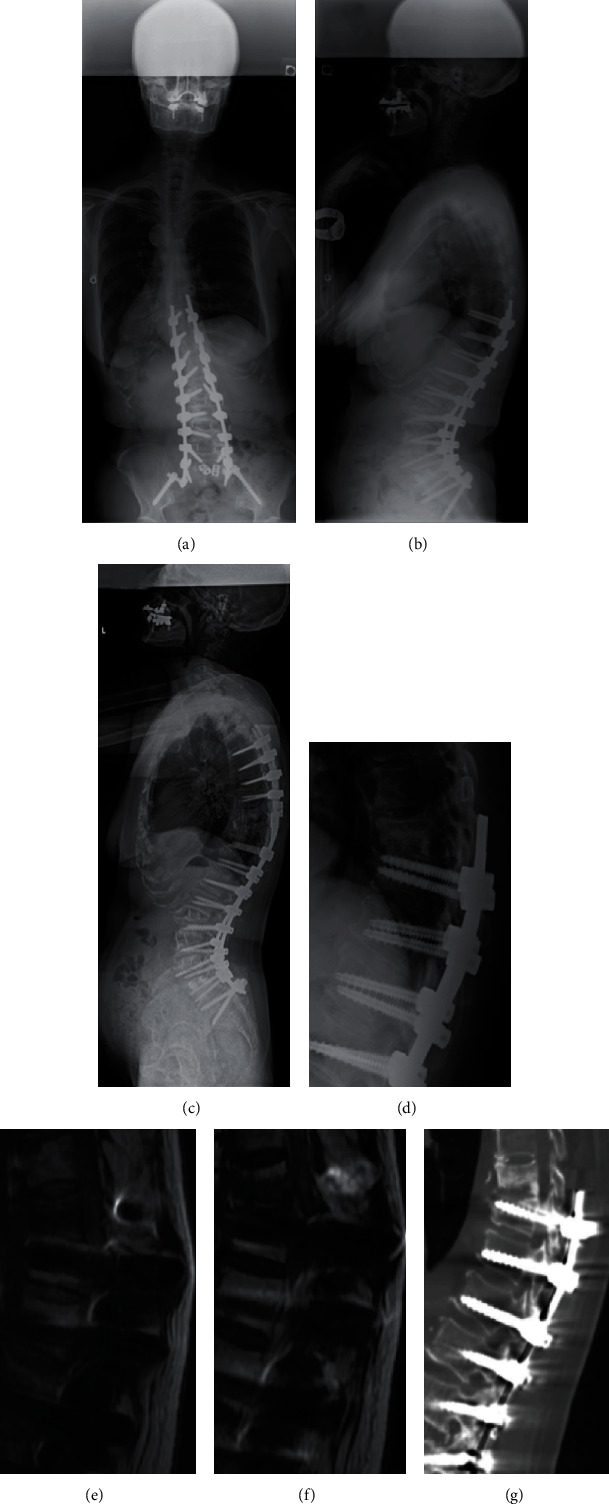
The 76-year-old female patient received PSF (Th10 to ilium). (a) Immediate postoperation whole spine PA standing radiograph demonstrates normal coronal alignment. (b) Immediate postoperation whole spine lateral standing radiograph demonstrates normal sagittal alignment. (c) Immediate postrevision whole spine lateral standing radiograph demonstrates normal sagittal alignment. (d) PA view at readmission shows moderate PJK (PJA 18.2 deg.). (e) T1WI around the upper instrumented vertebra (UIV) area shows bone destruction of the UIV vertebra. (f) T2WI around the UIV area shows fluid correction around the UIV+1 vertebra. (g) CT scan around the UIV area shows bone destruction of both UIV and UIV+1 vertebra.
